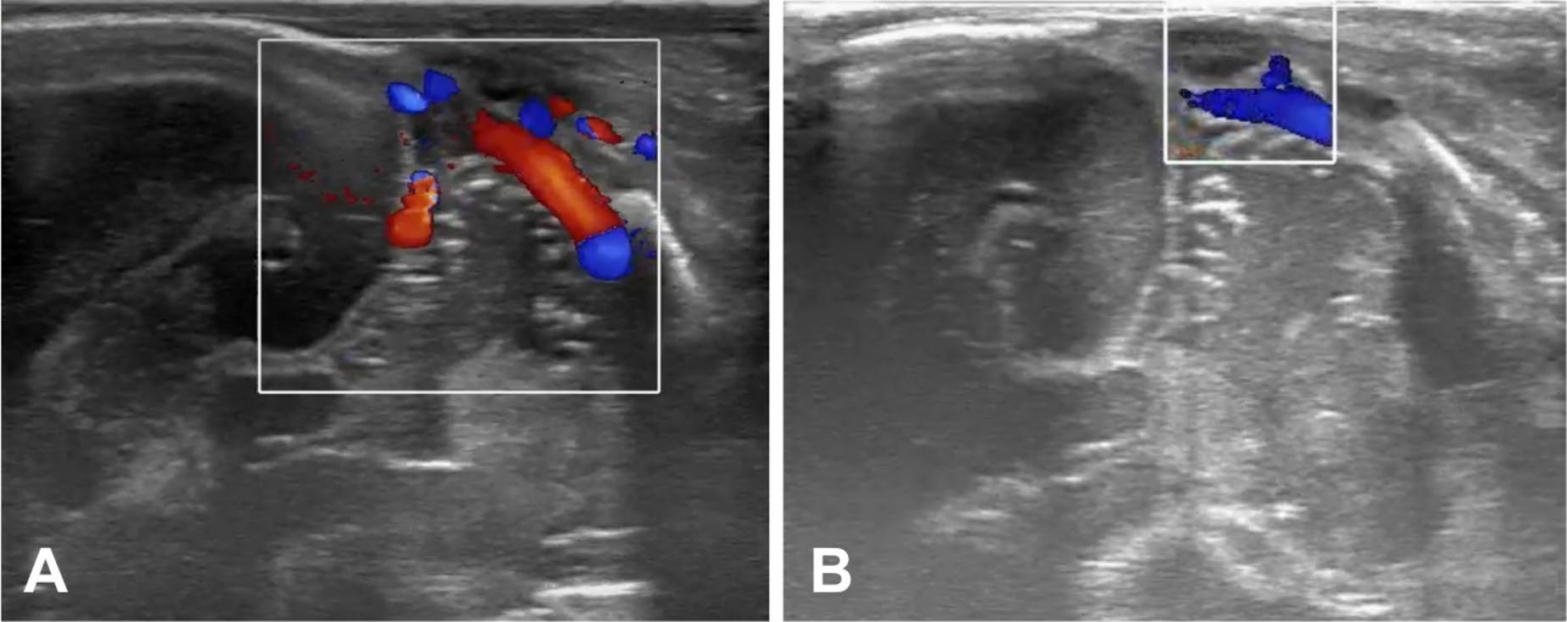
Cranial ultrasound imaging (recanalization). A Ultrasound images obtained during the exam on the 40th day of life (the day of recognition of thrombus resolution) on right. B Ultrasound images obtained during the exam on the 40th day of life (the day of recognition of thrombus resolution) on left side
